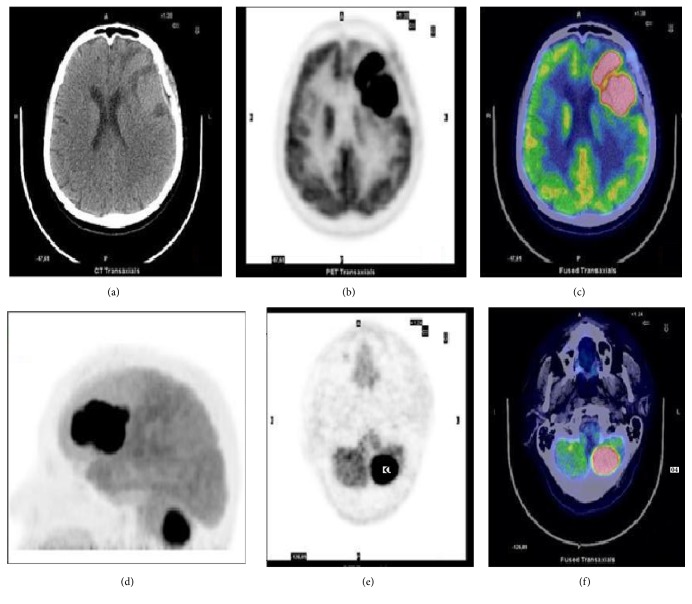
^18^F-FDG brain imaging in a 66-year-old woman with PCNSL. Axial CT (a), PET (b), and PET/CT fusion image (c) showing the FDG-avid lesion involving the left frontal lobe (SUVmax 42). (d) Maximum imaging projection (MIP), axial CT (e), and PET/CT fusion image (f) showing another lesion on the left cerebellar hemisphere.
